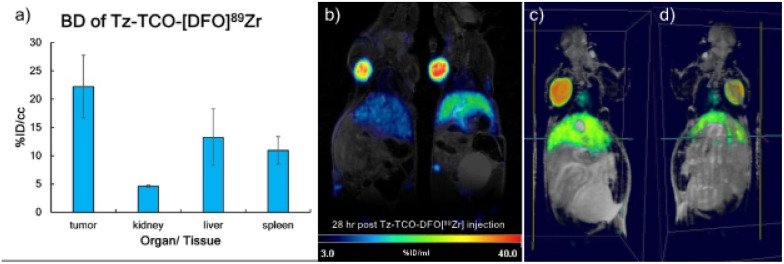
a) Biodistribution of Tz-TCO-DFO[^89^Zr], analyzed using PMOD software, normalized to the injection time point to take into consideration of radioactive decay. b) Co-registered PET-MRI images at 28 h after injection of Tz-TCO-DFO[^89^Zr]. Image fusion was done with PMOD software. c)-d) 3D PET-MR fused image. Volume-rendered images were generated with ParaVision Software.
